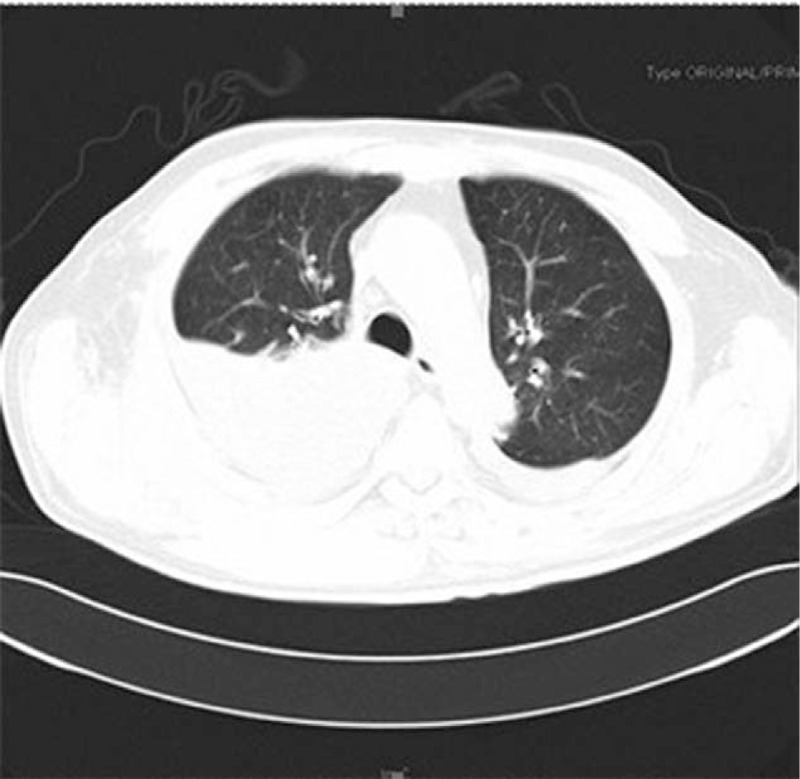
Computed tomography (CT) scan of chest revealed right-sided pleural effusion.
